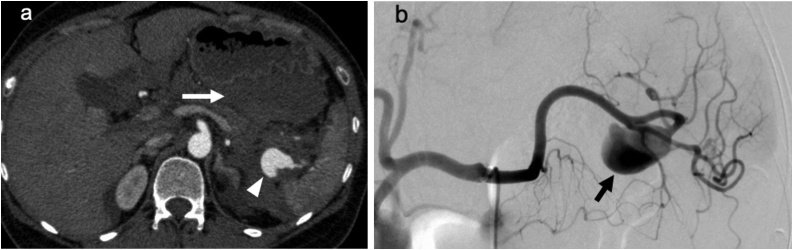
Computed tomography and angiography images. (a) Enhanced abdominal computed tomography showed a splenic artery aneurysm of 37 mm in diameter, which was located near the splenic hilum (arrowhead). Fluid collection was present around the splenic artery aneurysm, particularly in the lesser sac (arrow). (b) Selective angiography of the splenic artery showed the splenic artery aneurysm without apparent active extravasation (arrow). Multiple peripheral arteries were situated distal to the splenic artery aneurysm and might have been connected to the aneurysm.
